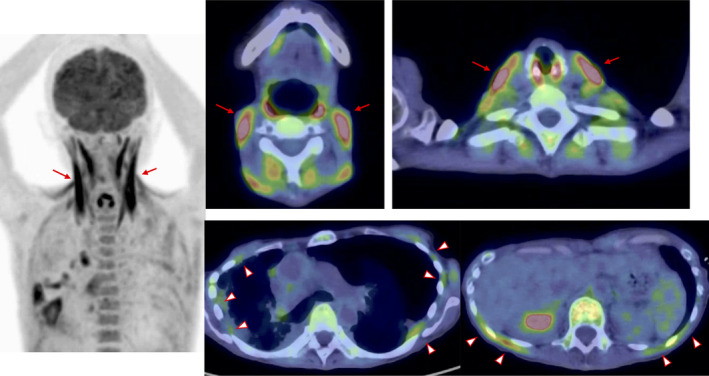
^18^F‐fluorodeoxyglucose (^18^F‐FDG) accumulated in cervical muscles and intercostal muscles (arrowheads). In particular, the uptake of ^18^F‐FDG in sternocleidomastoid muscles (arrows) was high, and the maximum standardized uptake value was 3.2–4.4
